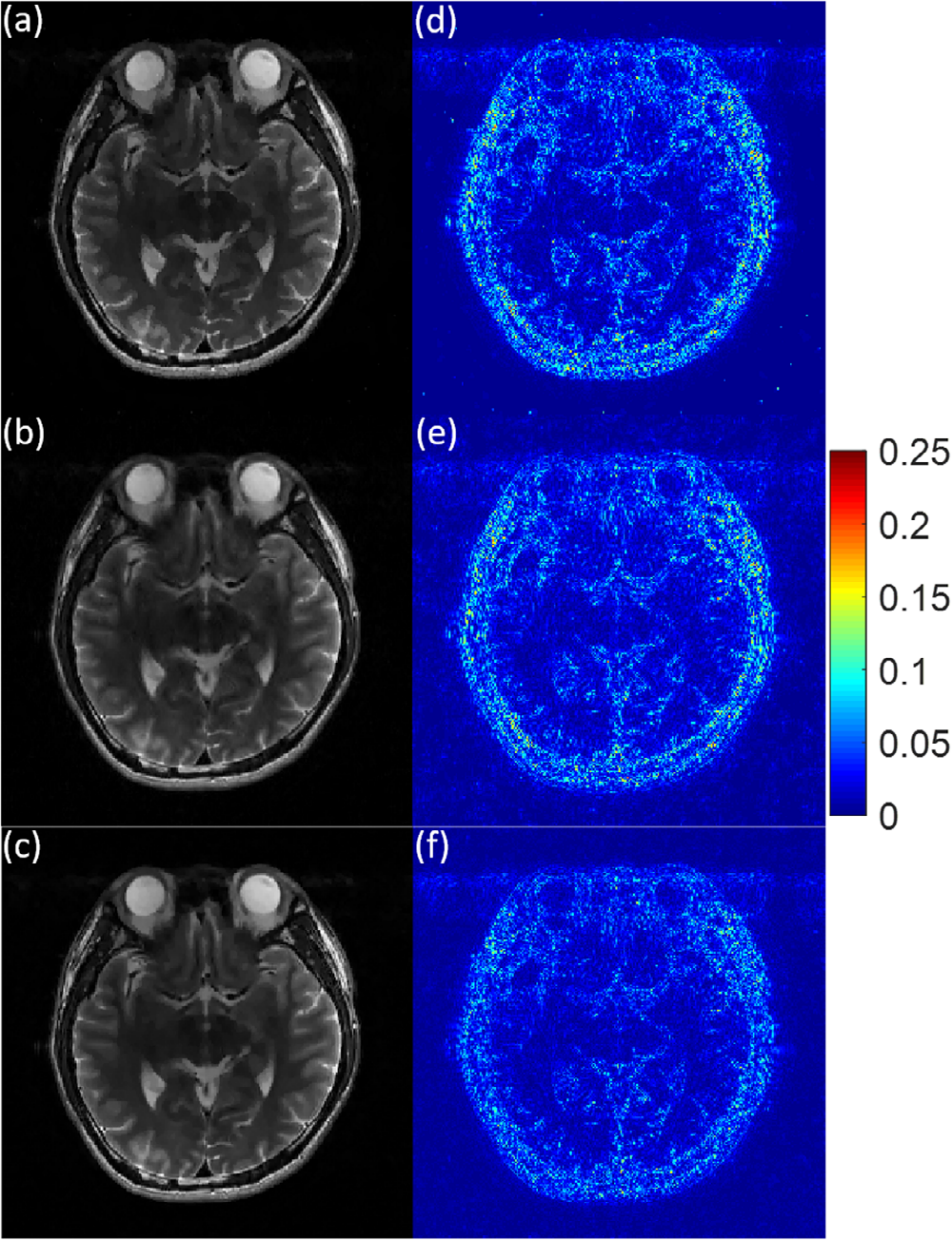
Reconstruction results of the 7th slice of the brain MRI 1. The parts of the figure (a)–(c) are the reconstruction results of MCTV‐L_2_, pFISTA, and TV+TFL_0_. The parts (d)–(f) are corresponding error images of (a)–(c).
